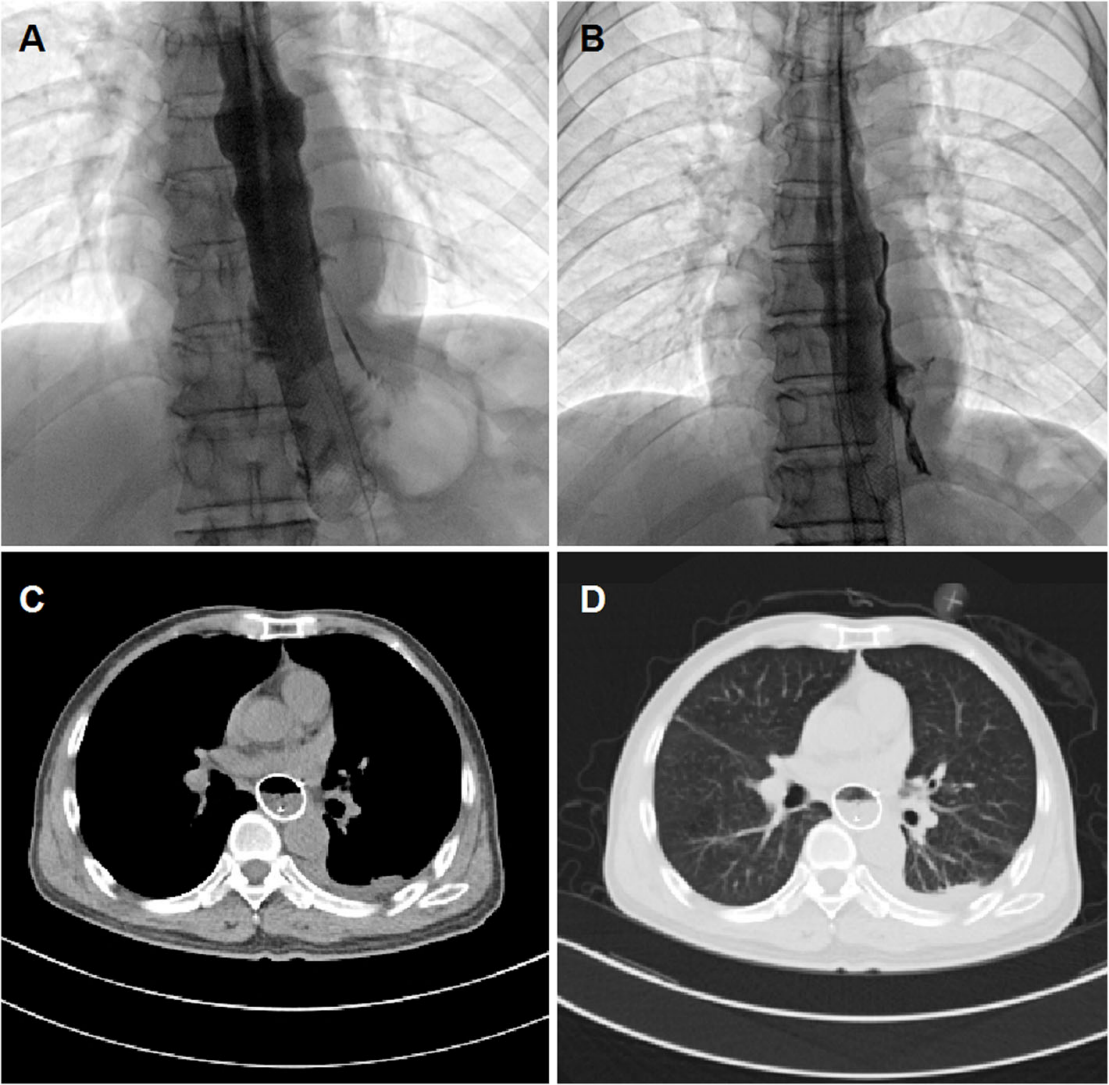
Three-tube method and covered stent placement for case 24. a After esophageal covered stent and drainage tube placement, esophagography shows that the contrast agent flows though the esophagus and stent with no leakage. b Esophagogram via drainage tube showing a decreased abscess cavity during follow up. c–d At 1.6 months after three-tube treatment and stent placement, a chest CT scan shows decrease in mediastinal abscess and pleural effusion
